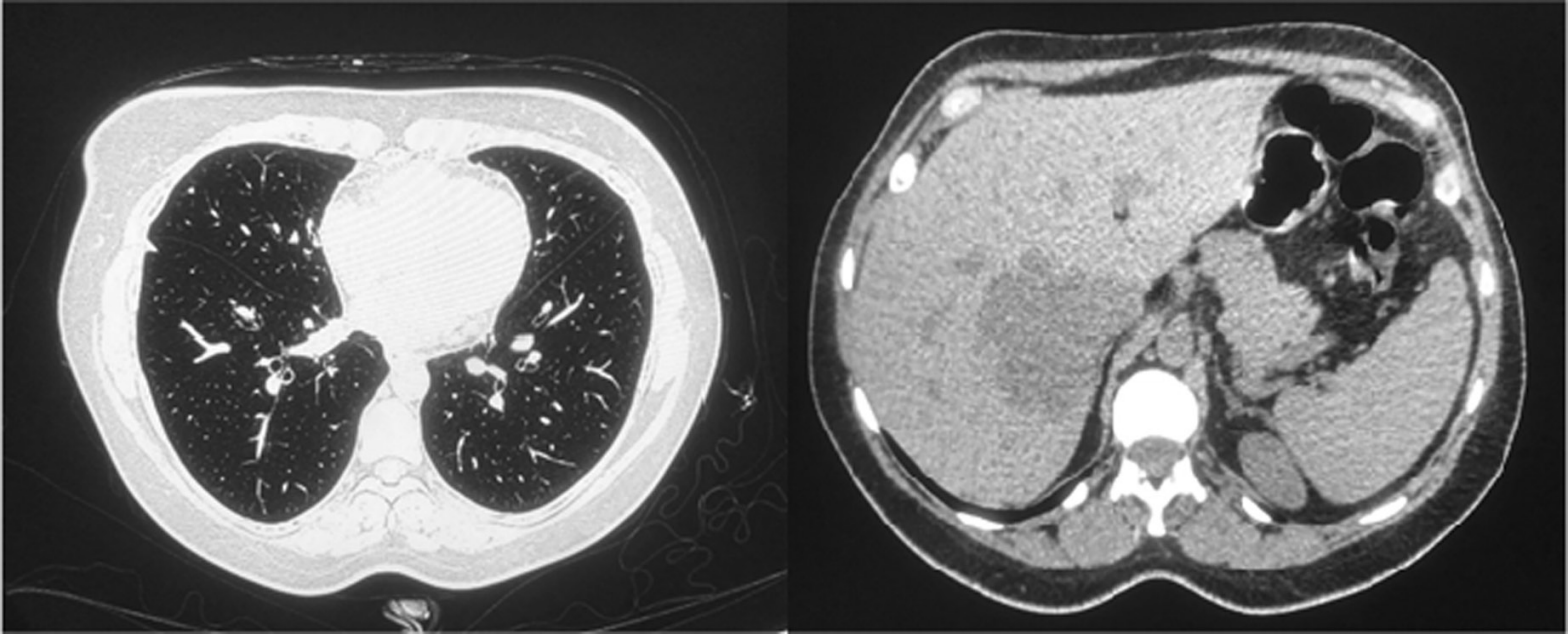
Chest CT scan with contrast for the patient.
